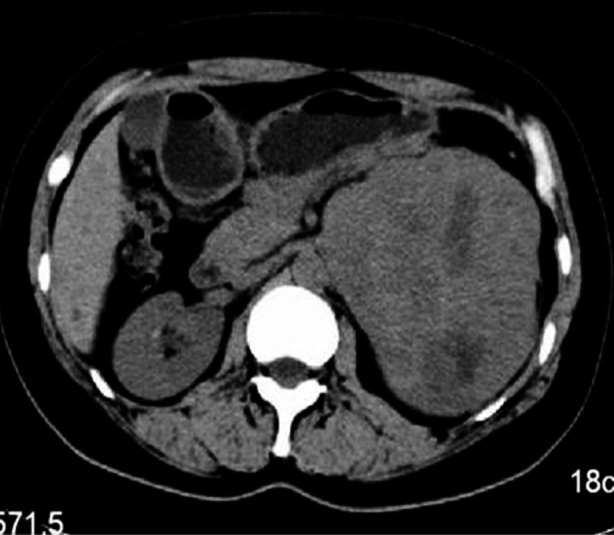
Plain CT manifestation of intra-abdominal DSRC. The tumor with 11.0 × 12.5 cm was in upper left abdomen, with clear boundary, uneven density, and patchy necrotic and cystic area within the tumor; the CT value was 36 HU.
